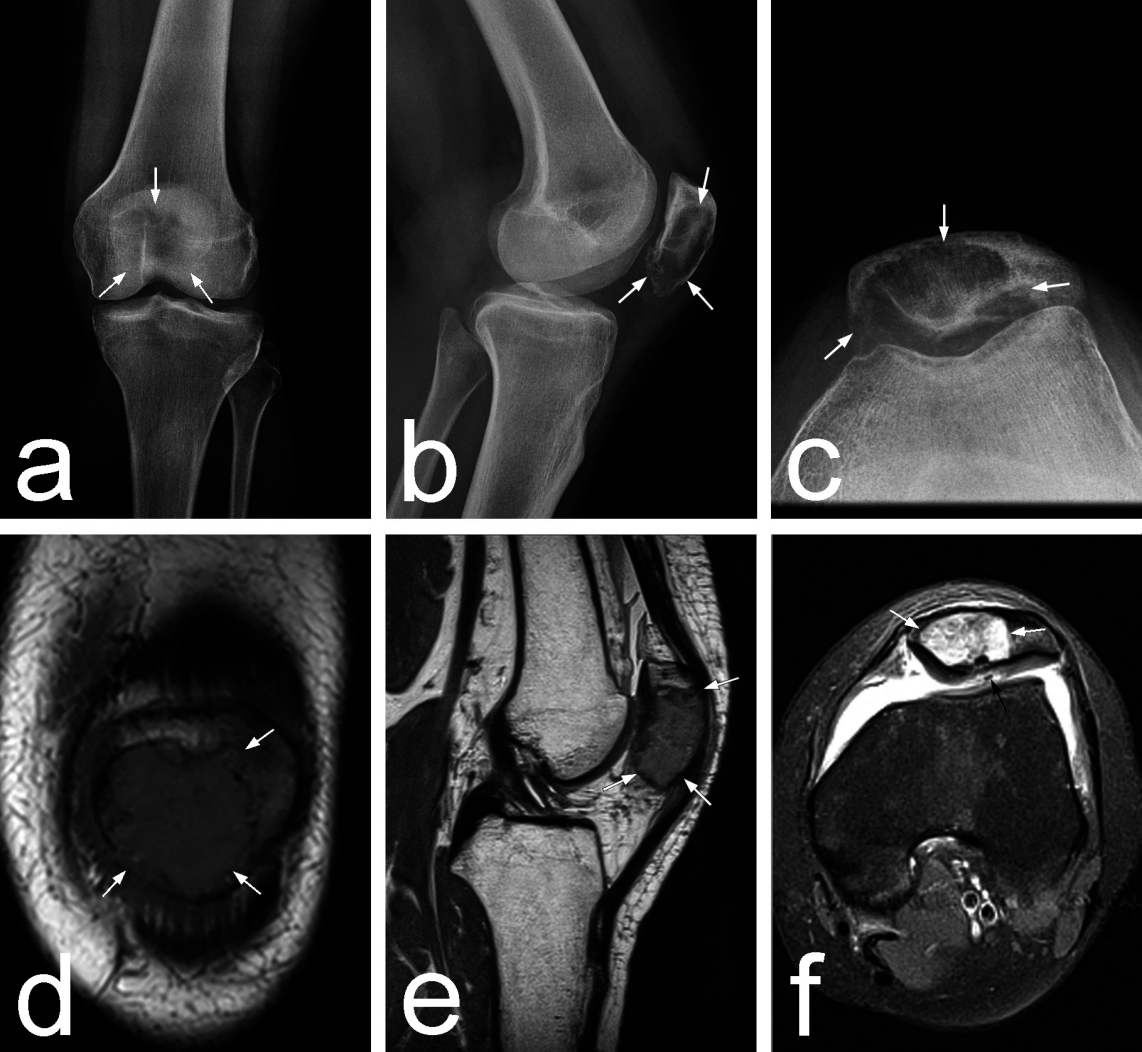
Plain radiographs and MRI at presentation 1A-1C: AP, lateral, and axial radiographs of the knee showing tumor extent (arrows). 1D-1F: Frontal, sagittal, and axial MRI slices trough the patella showing tumor extent (white arrows) and cartilage lesion (black arrow). MRI: magnetic resonance imaging
